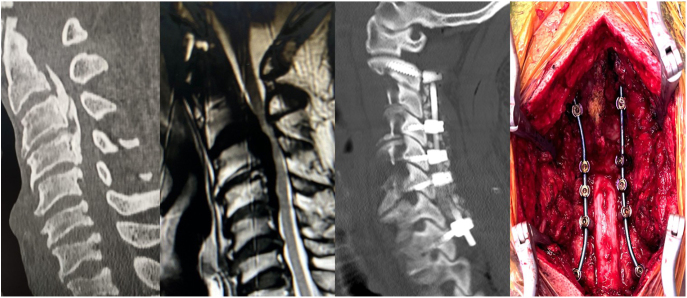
From the left, a cervical CT scan and MRI showing a C3-C4 massive OPLL. A surgical treatment consisting of laminectomy and C2-T1 fixation was performed.
